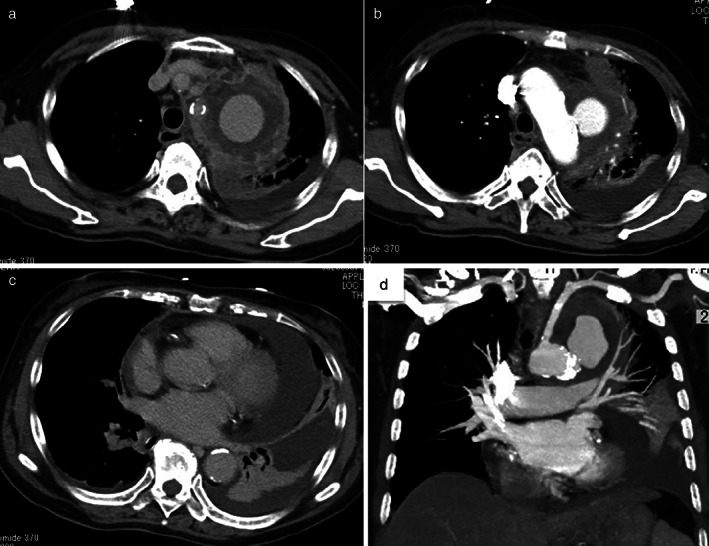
Results of urgent contrast‐enhanced computed tomography (CT) of the chest. (a–c) Axial images. (d) Sagittal images. Contrast‐enhanced CT of the chest reveals pseudoaneurysm of the descending thoracic aorta. Pleural effusion and pericardial fluid are worse than those from CT of the chest on admission.
